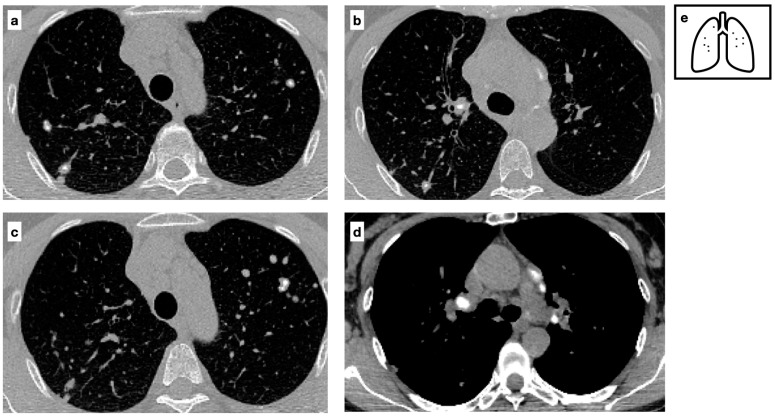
CT scans of a Patient with a diagnosis of sarcoidosis demonstrating multifocal nodules characterized by central calcifications (a–c) and calcified lymph nodes are present within the mediastinum (d). Mediastinal lymphadenopathy in sarcoidosis is typically bilateral and symmetric; lymph nodes may calcify over time, commonly exhibiting ‘popcorn’, amorphous and punctate calcifications. Pattern of calcification (e).
